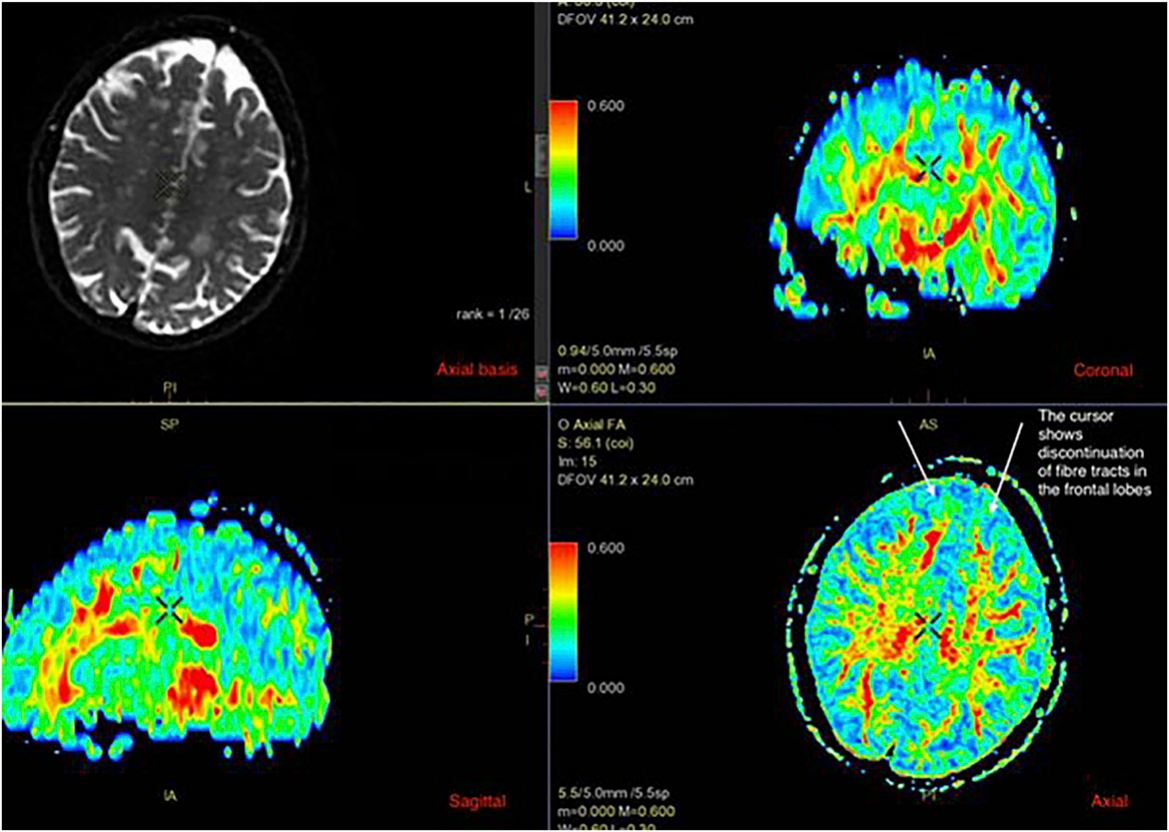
An example of diffusion tensor imaging MRI in a patient with dementia.
